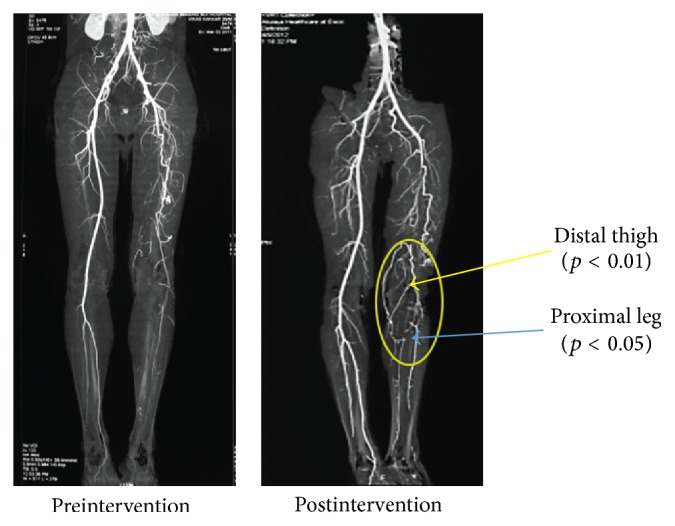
CT Angiography images showing qualitative analysis of degree of angiogenesis.
